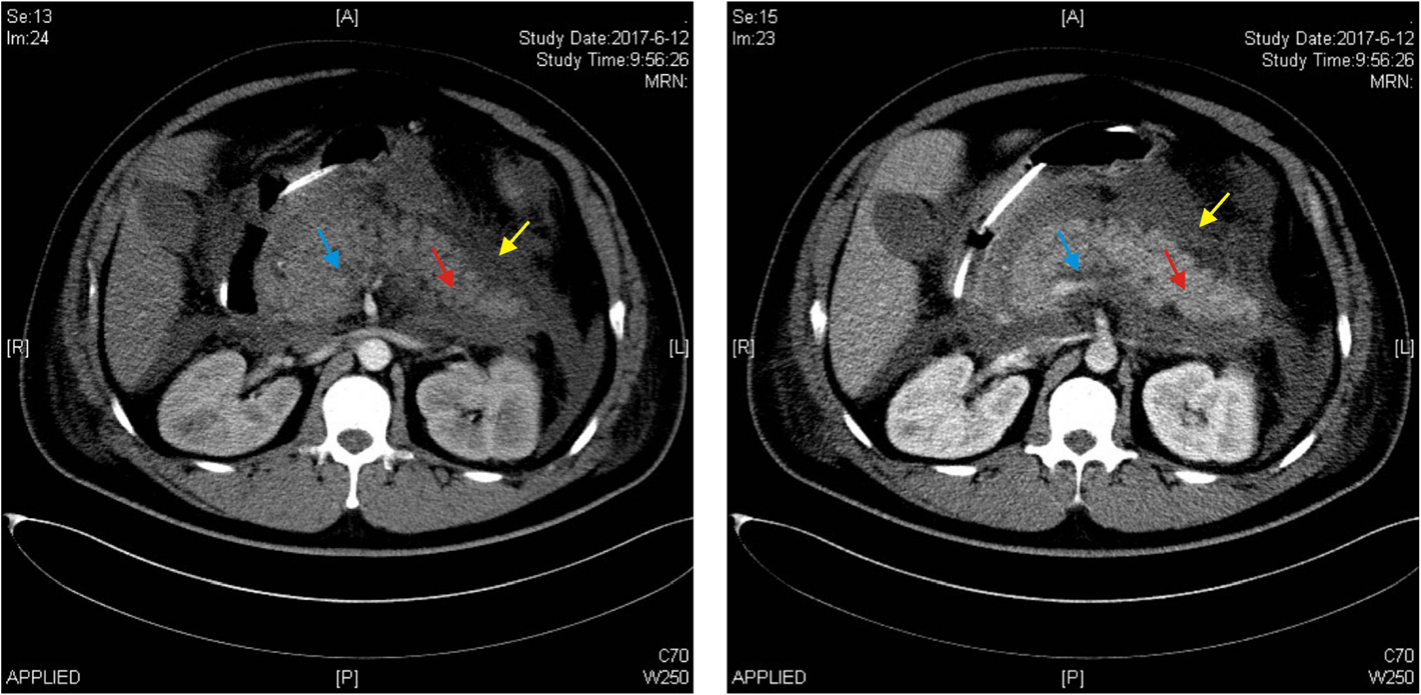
Abdominal computed tomography (CT) showing the enlarged pancreas with adjacent water density shadow, disappearance of the space among peripancreatic adipose tissues in the patient. Left panel, plain CT scan. Right panel, enhanced CT scan. Blue, red and yellow arrowheads indicate acute necrotic collection, pancreatic edema and acute peripancreatic fluid collection, respectively
